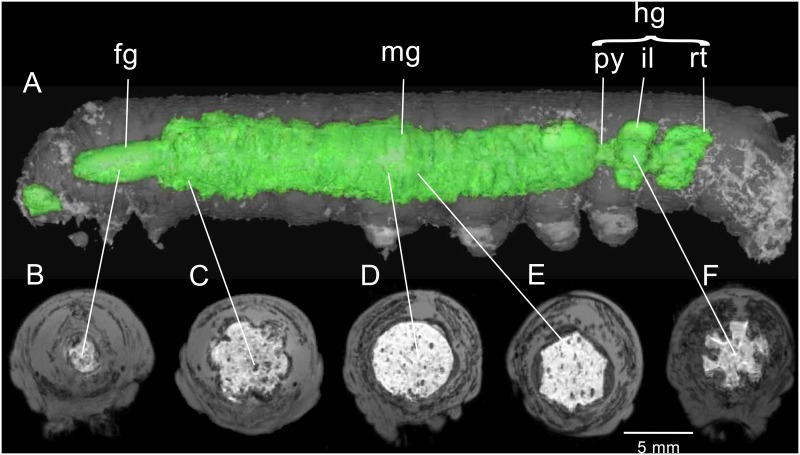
3D-GRE MR imaging data acquired from a 92 hour feeding fifth instar *M*. *sexta*. (A) Longitudinal surface-rendered view showing the digestive tract, including the foregut (fg), midgut (mg) and hindgut (hg), the latter consisting of the pylorus (py), ilium (il) and rectum (rt). Lack of CA between the buccal cavity and the esophagus may be due to swallowing. The insect has just defecated. White lines connect regions in the longitudinal view with corresponding transverse sections. (B) Foregut region. (C) Anterior midgut region, with prominent out-pocketings. (D) Middle midgut, displaying rounded lumen. (E) Middle midgut, displaying hexagonal-shaped lumen. (F) Hindgut, displaying the ileal nodes.
